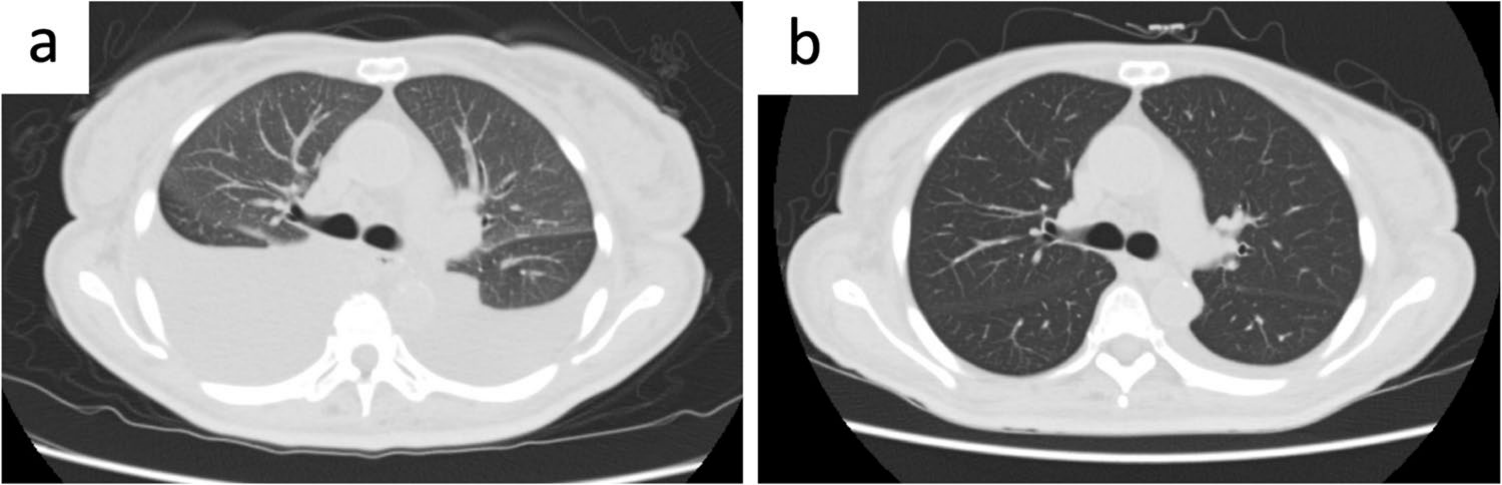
CT at admission (a) and on 12 days after admission (b)
